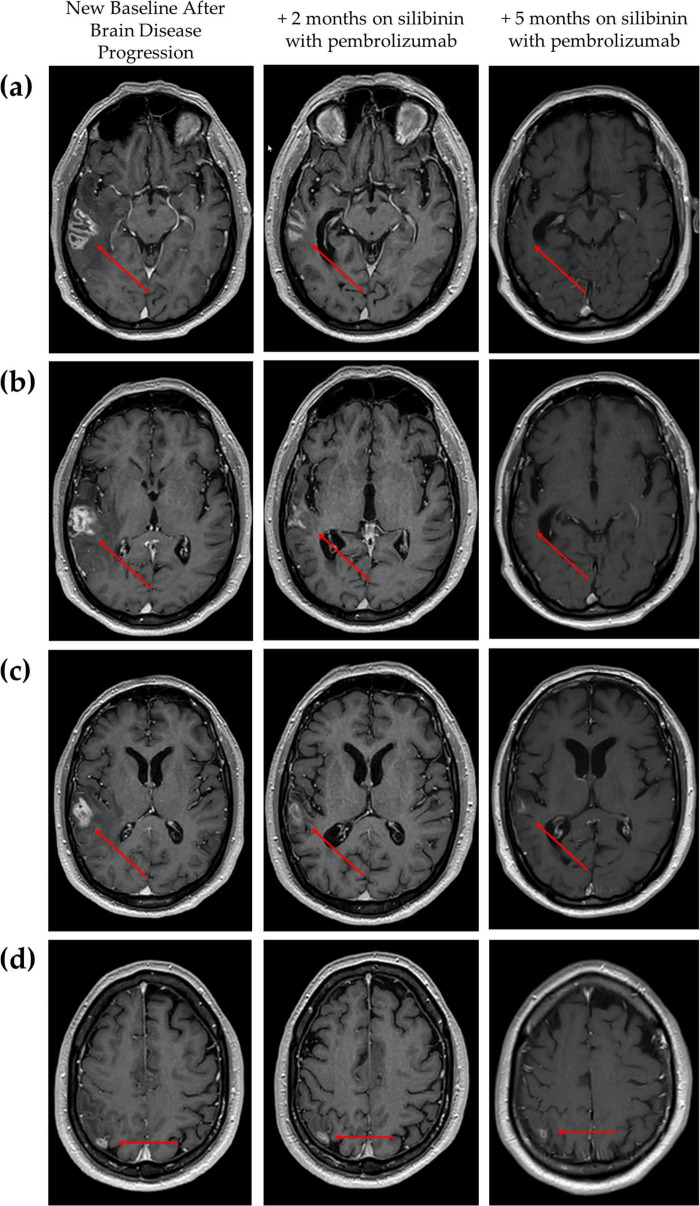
Brain magnetic resonance imaging (MRI) showing the radiological extension of the brain metastases at new baseline (when brain progression was confirmed on pembrolizumab) and evolution after silibinin supplementation with pembrolizumab at 2 and 5 months. (a–c) Radiological changes of right temporal lobe lesion over combination treatment using different slices to reflect its heterogeneous nature and (d) Radiological changes of right parietal lesion over combination treatment. Red arrows indicate the lesion.
